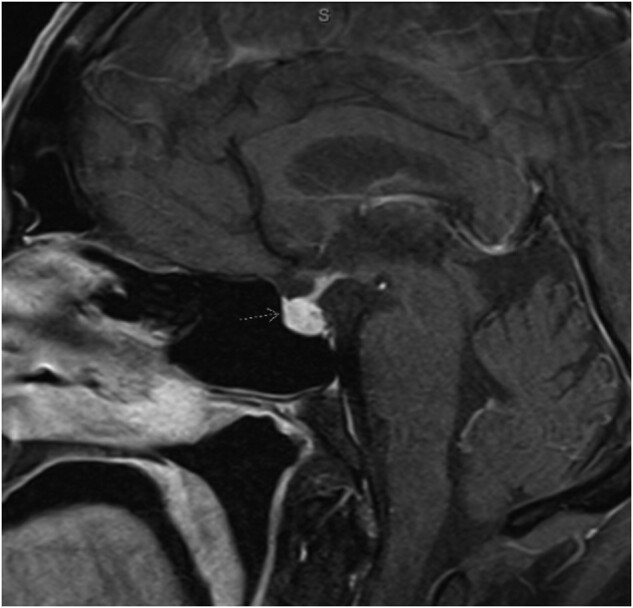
MRI while on pembrolizumab, showing new enlargement and homogeneous enhancement of the pituitary gland and infundibulum with convex superior margin.
